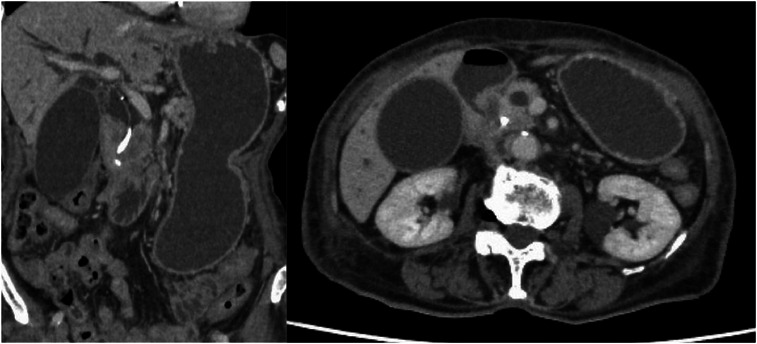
Computed tomography demonstrated an enlarged pancreatic head tumor causing a gastric outlet obstruction (GOO) and malignant biliary obstruction. The stomach and intrahepatic bile ducts were dilated.
